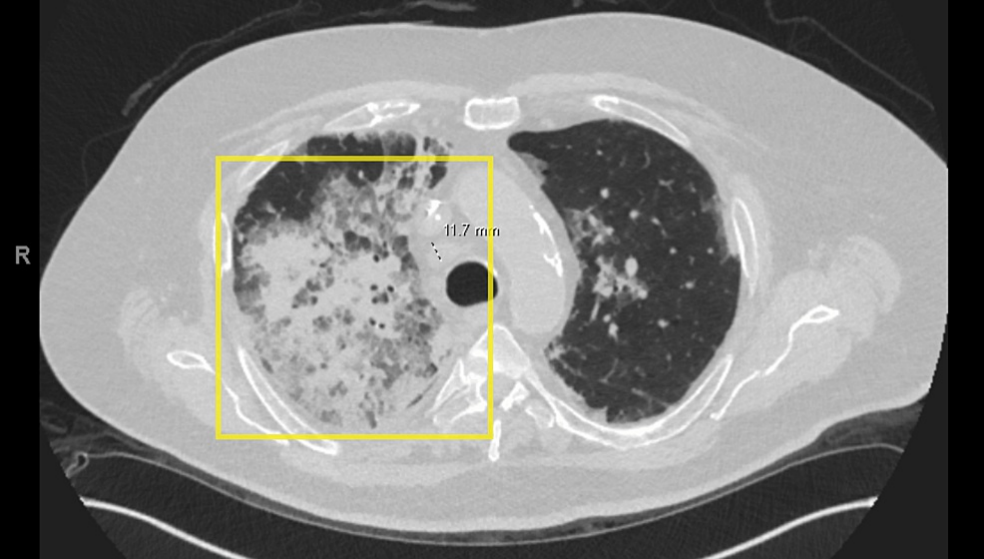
Computed tomography of the chest without contrast showing extensive right-sided infiltrates (yellow box) and hilar adenopathy (dotted black and white line) with smaller left-sided infiltrates
